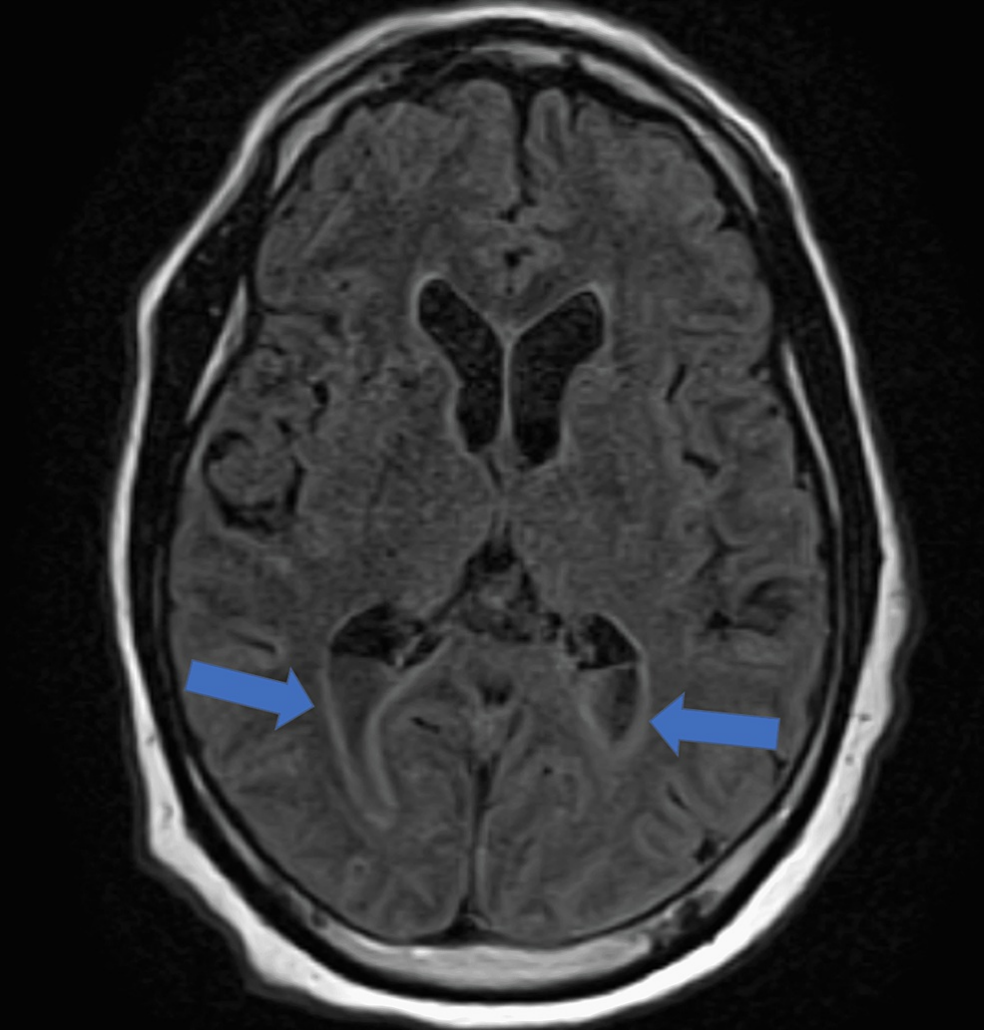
MRI of the brain demonstrating ventriculitis with thickening of the subependymal lining along the lateral ventricles and pus layering within the ventricular system
